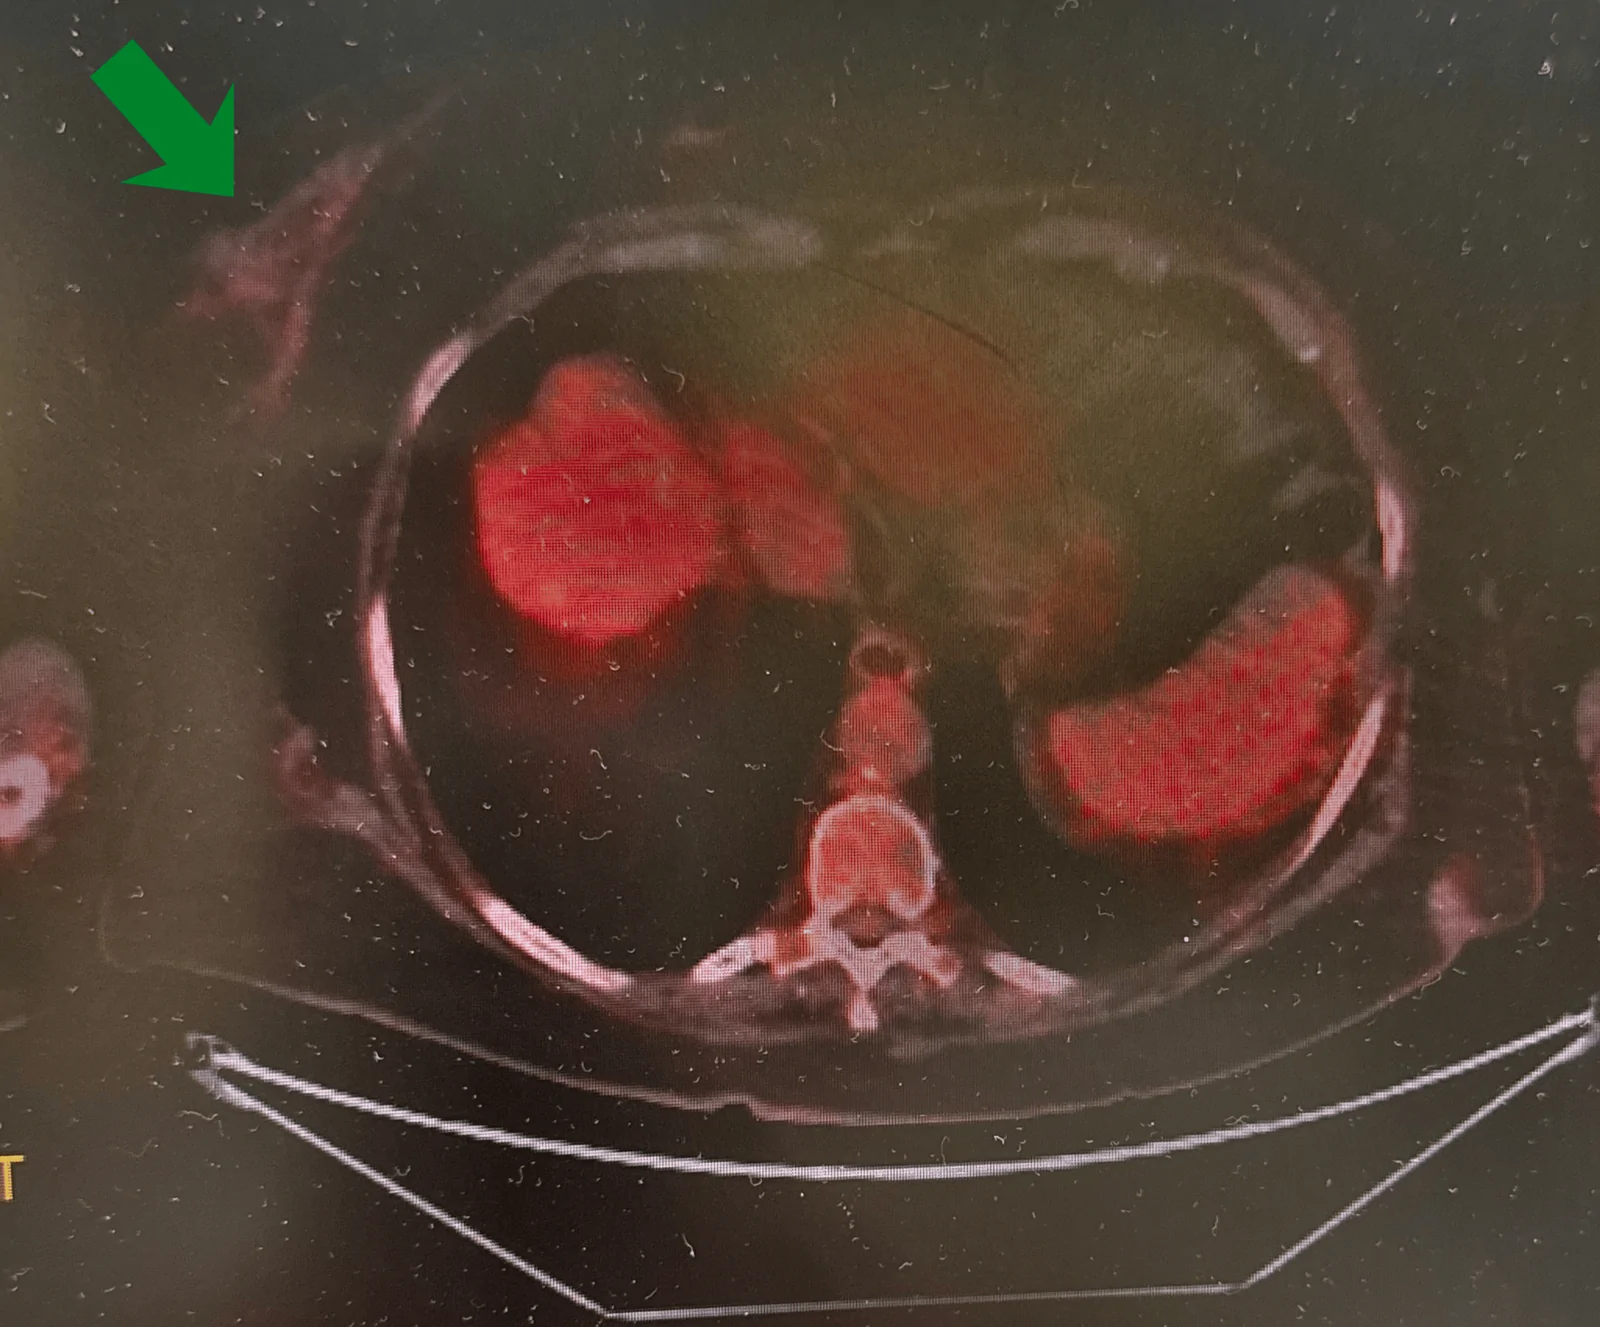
18F-FDG PET/CT demonstrating FDG-avid right breast malignancy Axial fused PET/CT image demonstrates an area of increased FDG uptake corresponding to the newly identified right breast lesion (green arrow). 18F-FDG: fluorine-18 fluorodeoxyglucose, PET/CT: positron emission tomography/computed tomography
